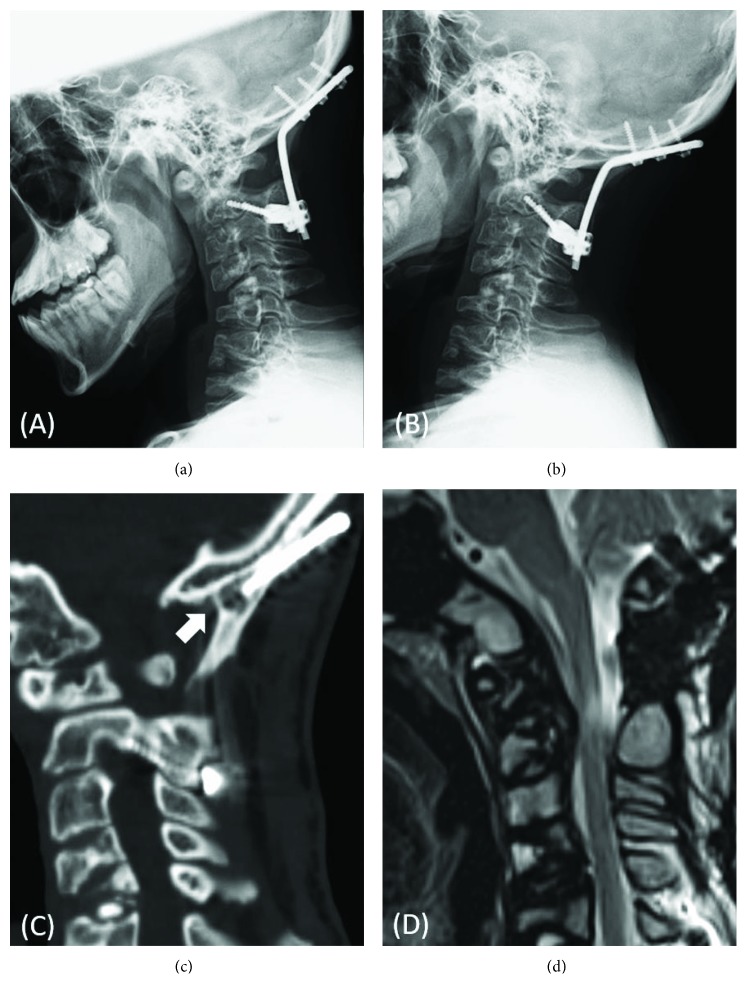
(a, b) Lateral radiographs of the cervical spine in flexion and extension at 1 year after surgery demonstrating a stabilized O-C2 segment. (c) Sagittal reconstruction computed tomography taken at 1 year after surgery showing solid fusion between the grafted iliac bone and occipital bone (*white arrow*). (d) Sagittal T2-weighted magnetic resonance image taken at 1 year after surgery revealing a well-decompressed spinal cord with marked regression of the retroodontoid pseudotumor.
